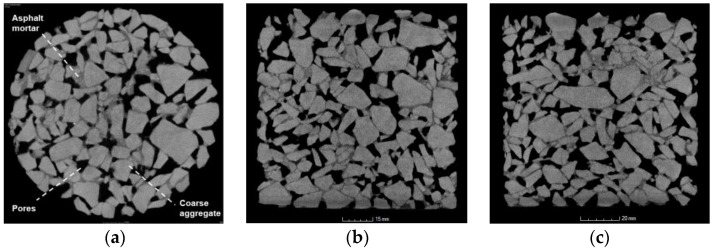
Original CT cross-sectional image: (a) plan view; (b) side view 1; (c) side view 2.
